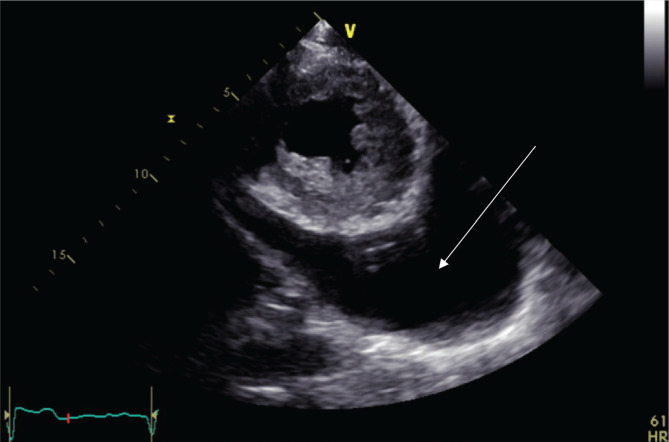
Echocardiography, short parasternal view.
